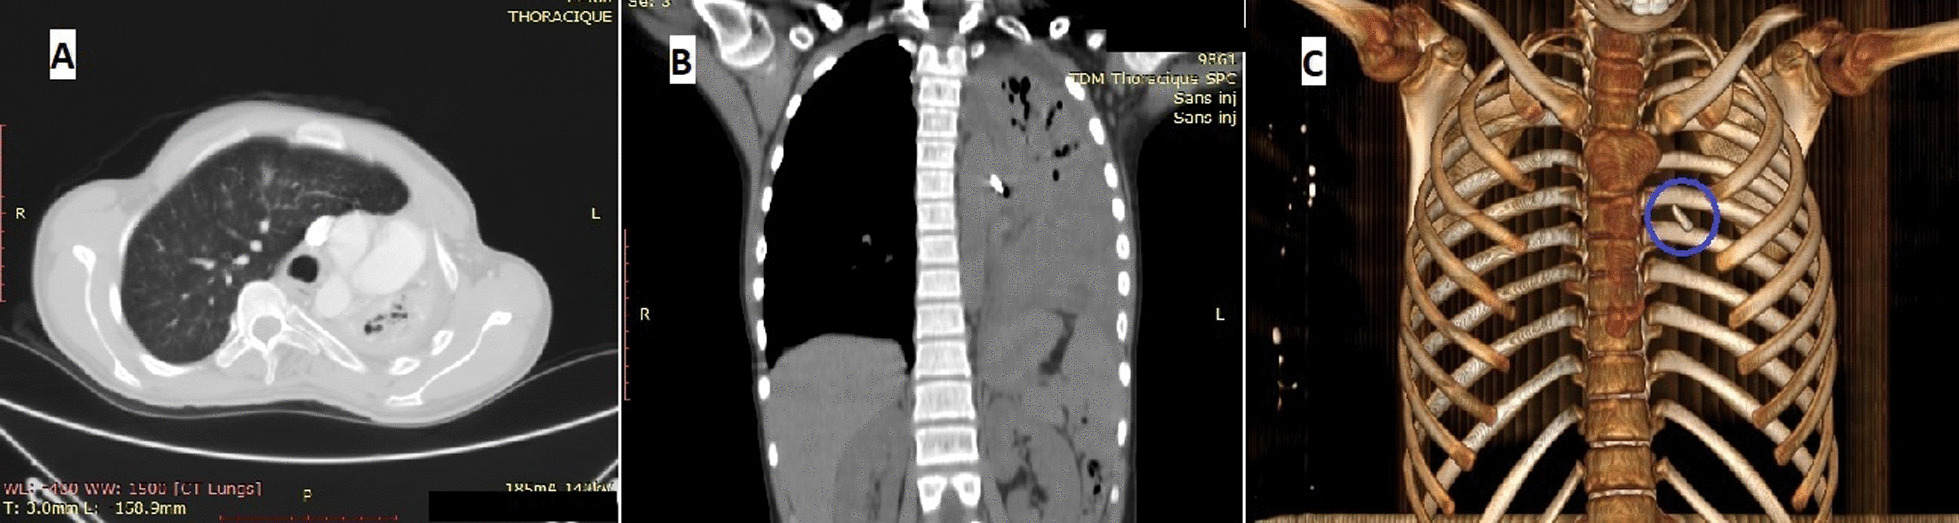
A Chest computed tomographic scan showing cavitary lesions in the left lung, as well as right lung herniates into the left chest. B, C Image of foreign body on thoracic computed tomography. The circle denotes a foreign body on thoracic computed tomography
